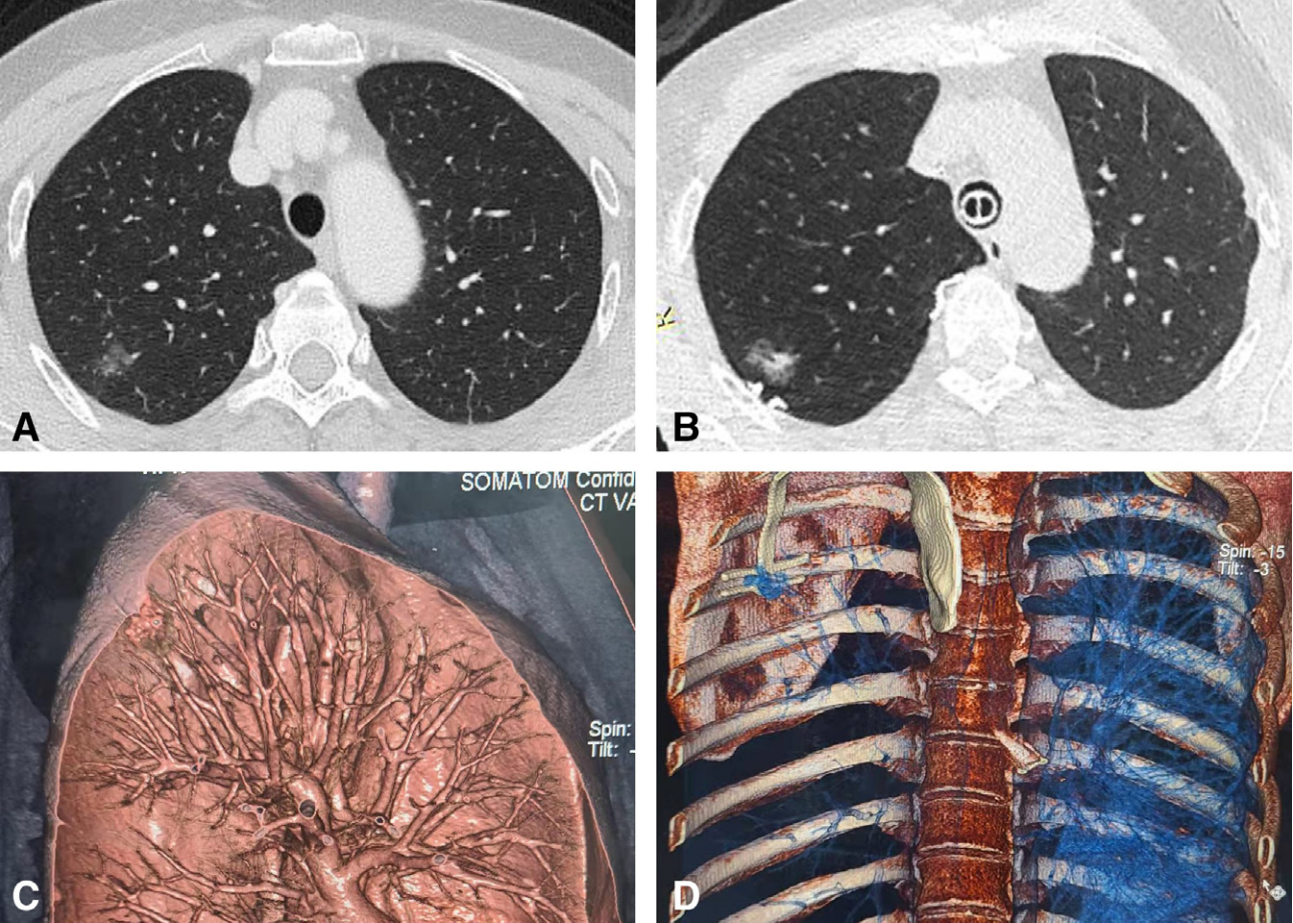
The precision of template-guided localization evaluated by intraoperative CT scanning. A, CT scan before template placement. B, Intraoperative CT scan after template placement. C, Location of nodules under 3D modeling. D, Location of the template under 3D modeling.
